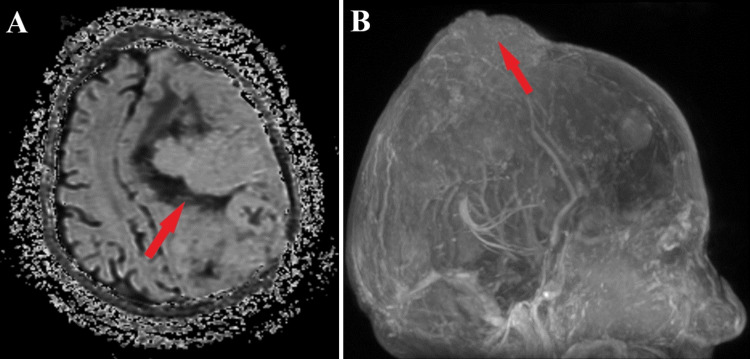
Preoperative ADC imaging aspect and 3D reconstruction Apparent diffusion coefficient (ADC) imaging scan (A) reveals the characteristic aspect of a malignant meningioma (red arrow), and a further 3D reconstruction (B) of MRI findings shows the extracranial morphological topography (red arrow).
